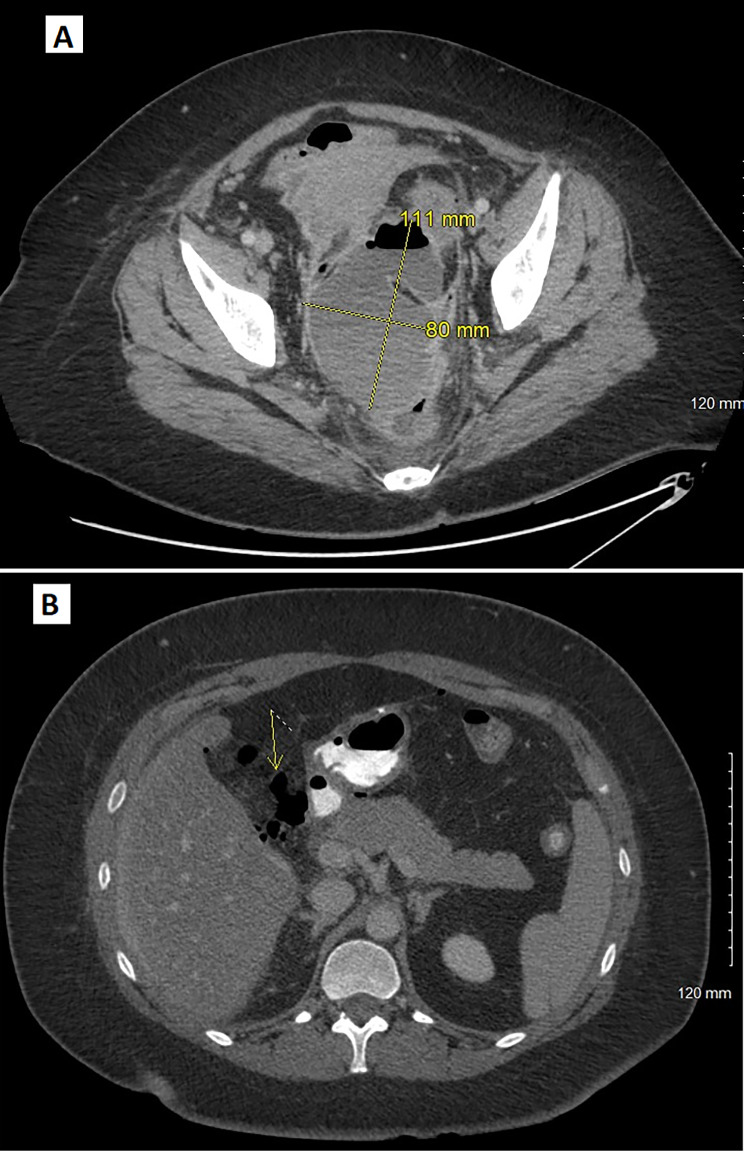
Major post-operative complications. a: 45yo patient, PO day-20, CT scan showed a pelvic collection compatible with infected hematoma (Dindo-Clavien IIIa). b: 34yo patient, PO day-1, CT scan showed a proximal duodenal leak (Dindo-Clavien IIIb)
